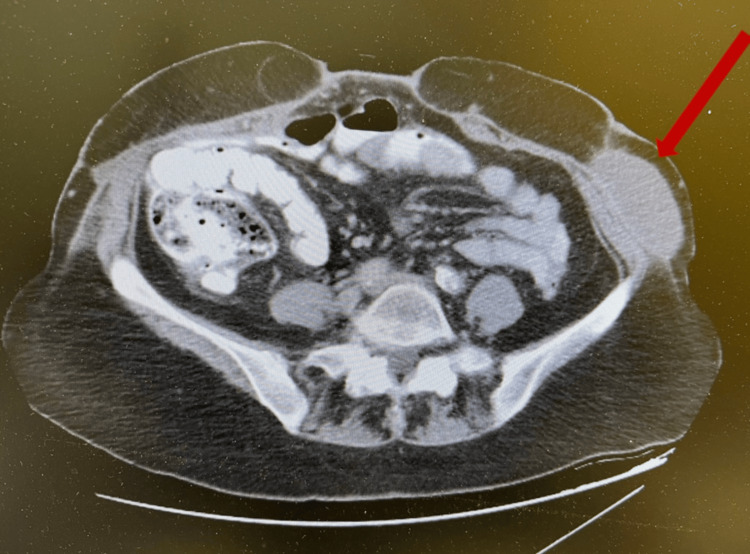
CT scan showing abdominal wall abscess and necrosis Abdominal wall abscess (red arrow) CT: computed tomography
